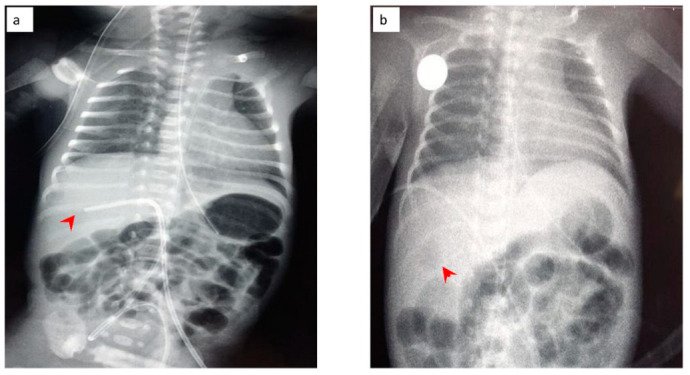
(a) Chest and abdominal radiography depicting the abnormal position of umbilical venous catheter in the liver (red arrowhead); and (b) chest and abdominal radiography depicting air in the branches of right portal vein and hepatic parenchyma (red arrowhead).
